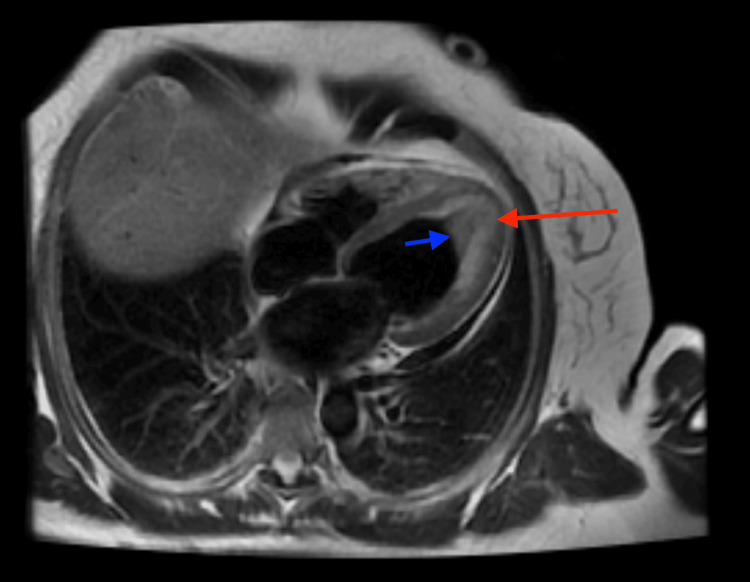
Cardiac MRI (T2 imaging, 4-chamber view). T2 weighted image demonstrates areas of hyperintensity in the left ventricular apex suggestive of underlying edema and inflammation (red arrow) resulting in complete obliteration of the apex. The overlying area of hypointensity was suspicious for an organized apical thrombus (blue arrow). T1 weighted image demonstrated significant late gadolinium enhancement of the left ventricular apical cavity consistent with a background of myocardial fibrosis and scarring (not depicted).
